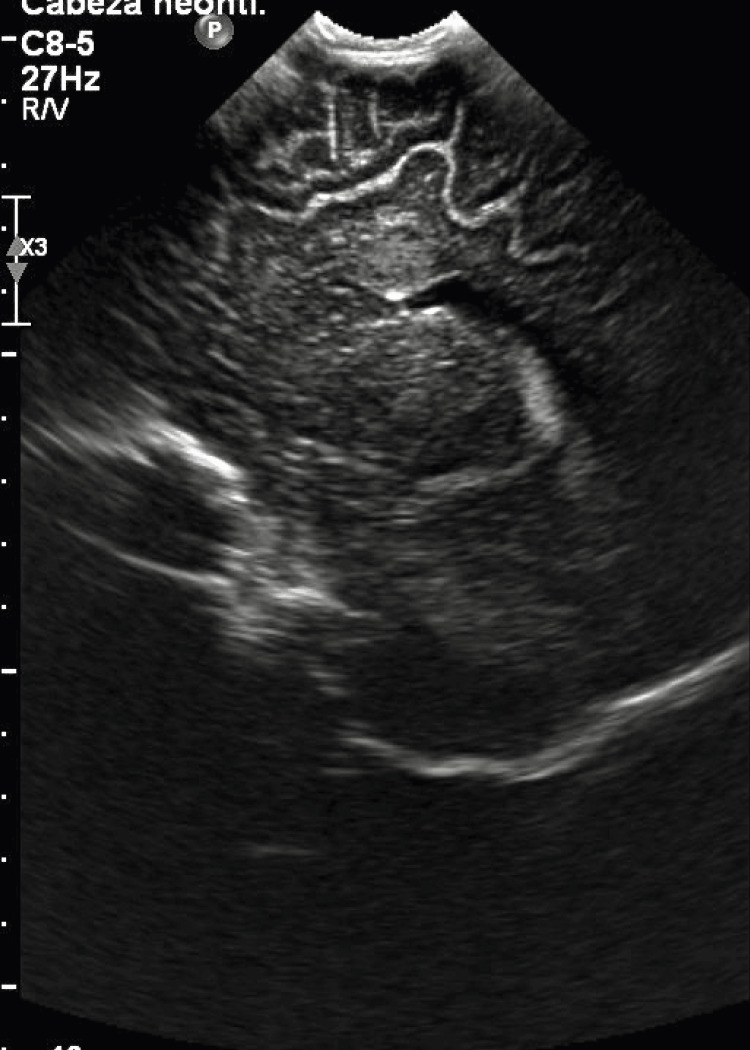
Image of the brain ultrasound showing a rounded image at the level of the roof of the right lateral ventricle of a five-month-old infant.
